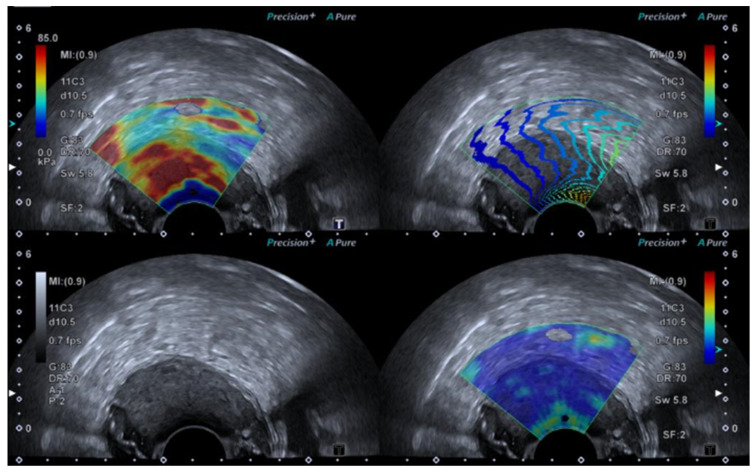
SWE ultrasound showing a t3b tumour in a 75-year-old male.
